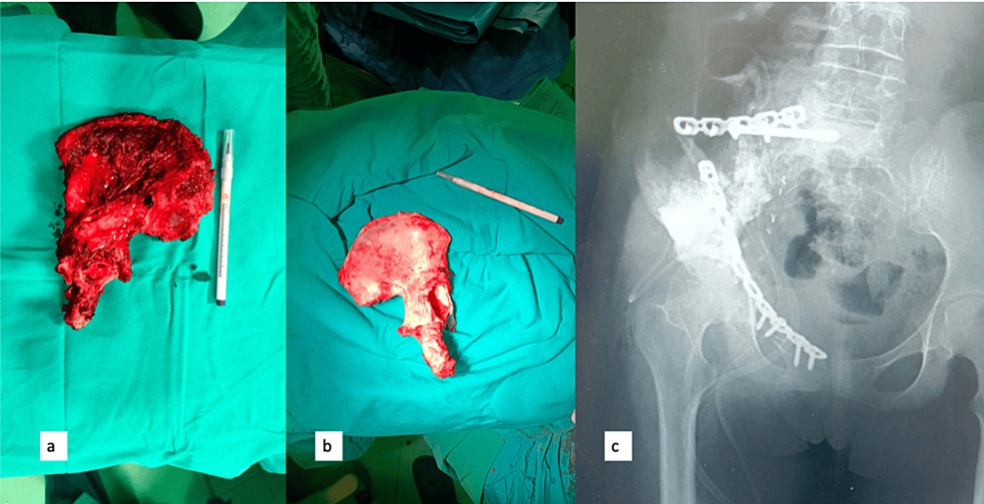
Clinical pictures of Ewing sarcoma patient using liquid nitrogen and biologic reconstruction; (a) clinical picture after tumor removal; (b) the prepared graft after the bone is cleared from tumor and liquid nitrogen treatment; (c) post-operative x-ray
